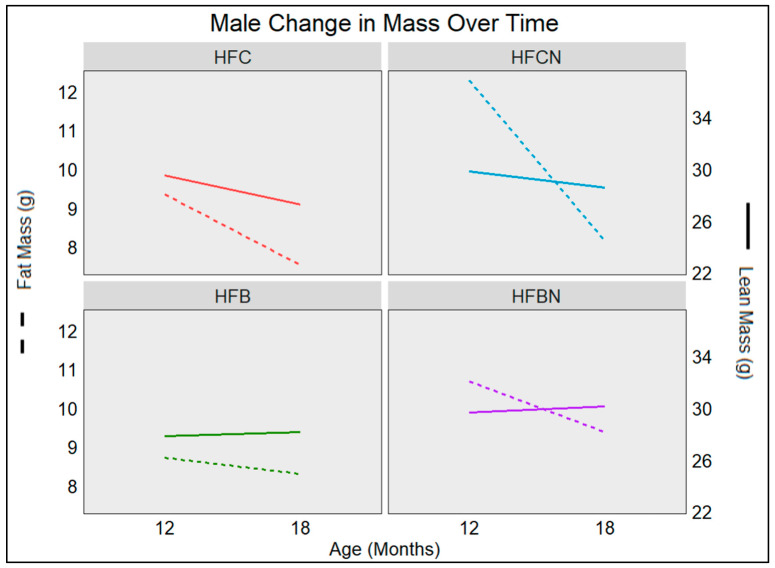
Visualization of changes in mass retention as the result of aging in C3H males. Measurements were taken at 12 and 18 months by Echo MRI. Solid lines represent change in lean mass (right *y*-axis), and dashed lines represent change in fat mass （left *y*-axis). Sample sizes (*n* > 4) and SE are reported in Table 2 for males.
